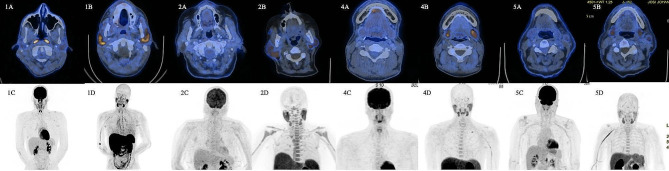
1A-1D: Axial hybrid FDG-PET/CT (1A), axial hybrid choline-PET/CT (1B), maximum intensity projection (1C) on FDG-PET, and maximum intensity projection of choline-PET (1D) of patient 1 with high-grade mucoepidermoid carcinoma of left parotid 2A-2D: Axial hybrid FDG-PET/CT (2A), axial hybrid choline-PET/CT (2B), maximum intensity projection (2C) on FDG-PET, and maximum intensity projection of choline-PET (2D) of patient 2 with low-grade acinic cell carcinoma of right parotid 4A-4D: Axial hybrid FDG-PET/CT (4A), axial hybrid choline-PET/CT (4B), maximum intensity projection (4C) on FDG-PET, and maximum intensity projection of choline-PET (4C4D) of patient 4 with adenoid cystic carcinoma of left submandibular gland 5A-5D: Axial hybrid FDG-PET/CT (5A), axial hybrid choline-PET/CT (5B), maximum intensity projection (5C) on FDG-PET, and maximum intensity projection of choline-PET (5D) of patient 5 with pleomorphic adenoma of left parotid gland
